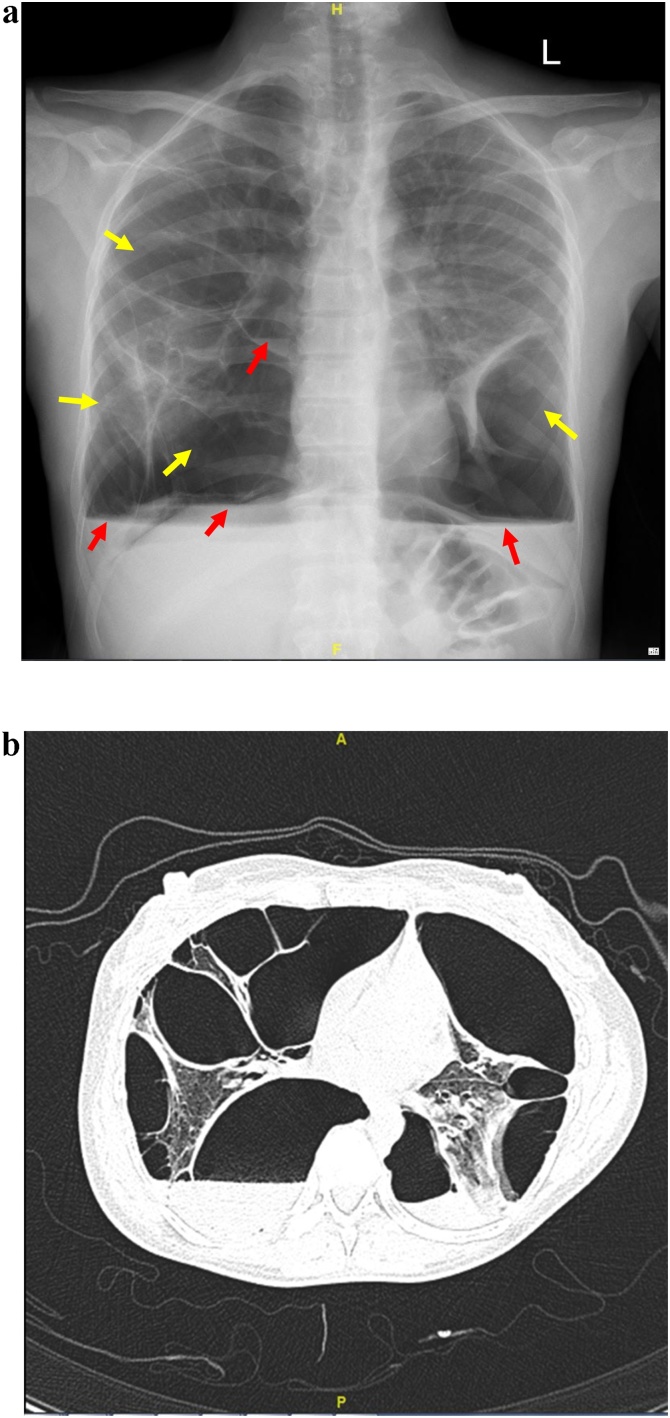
a) PA and lateral chest radiograph demonstrate numerous thin walled air containing structures/bullae some shows air fluid level. Yellow arrows: bullae. Red arrows: Air fluid level. b) Axial chest CT scan in lung window showing numerous air containing structures/bullae compressing underlying lung parenchyma with some shows air fluid level. No lung nodules. No bronchial wall thickening. No intra-thoracic liver herniation. Yellow arrows: bullae. Red arrows: Air fluid level.
